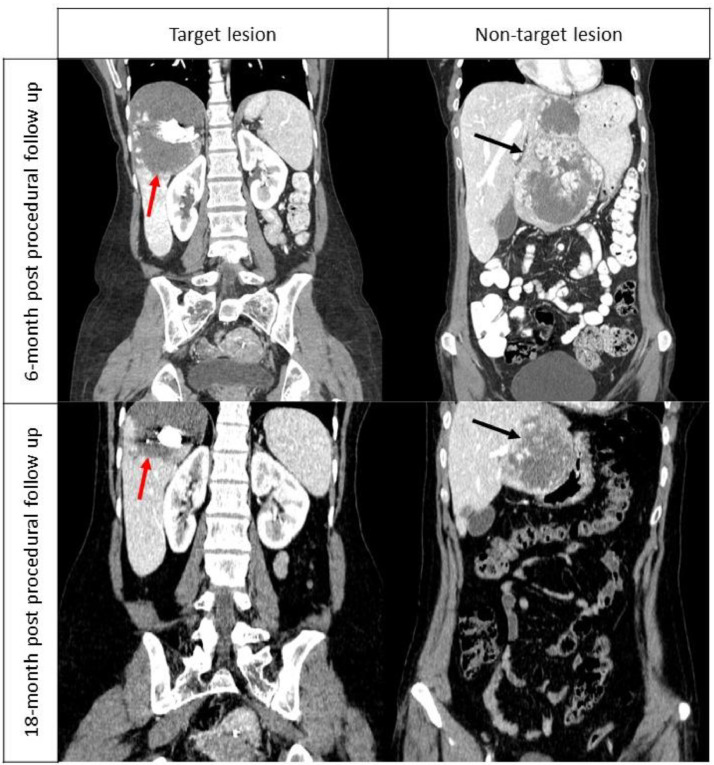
Multiphasic CT scan of the Case 2 in portal venous phase showing significant reduction in size of both target (red arrows) and nontarget (black arrows) hepatic hemangiomas, at 6-month (upper row) and 18-month (lower row) post-DS follow-ups (smallest lesion not shown).
